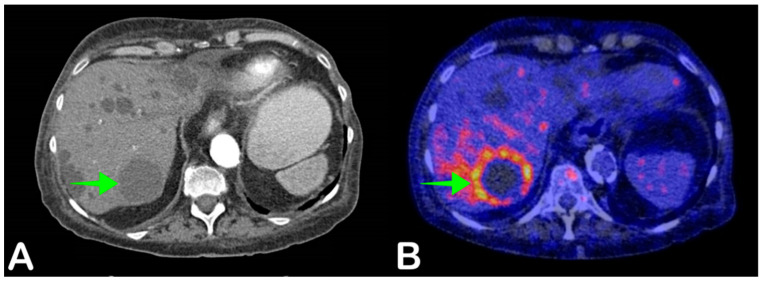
A 68-year-old woman presented with general malaise and night sweats for two weeks. She did not have a fever. She had received a kidney transplant 20 years earlier due to kidney failure caused by autosomal dominant polycystic kidney disease (ADPKD). Blood cultures were positive for *Escherichia coli*. Abdominal CT showed multiple liver cysts consistent with ADPKD, but no obvious signs of infection (A, green arrow). Fused axial FDG-PET/CT demonstrated increased FDG uptake in a large cyst in liver segment VII, suggestive of infection (B, green arrow). Antibiotic therapy was started with ciprofloxacin, after which the patient recovered.
